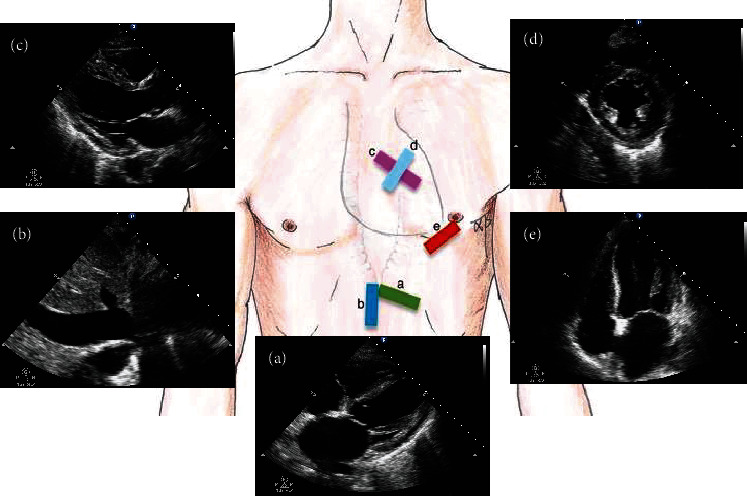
Five standard views used in critical care ultrasonography (CCUS). Subxiphoid long-axis (SLAX) view (a), subxiphoid inferior vena cava (SIVC) view (b), parasternal long-axis (PLAX) view (c), parasternal short-axis (PSAX) view (d), and apical four-chamber (A4CH) view (e).
